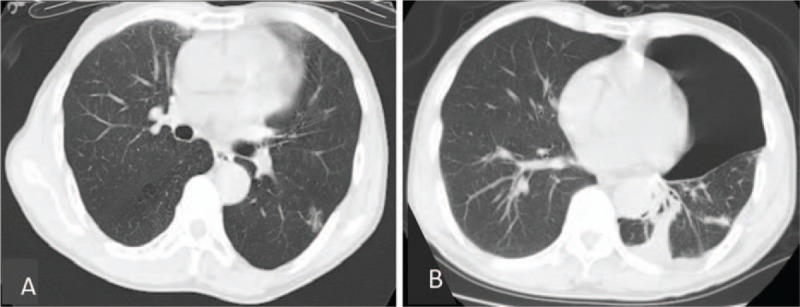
(A) Before treatment, CT-scans revealed a round mass with ground glass opacities in the lower left lobe of the lungs. (B) After surgery, the CT-scan displayed the shadow of the left lower lung wedge resection. CT= computed tomography.
